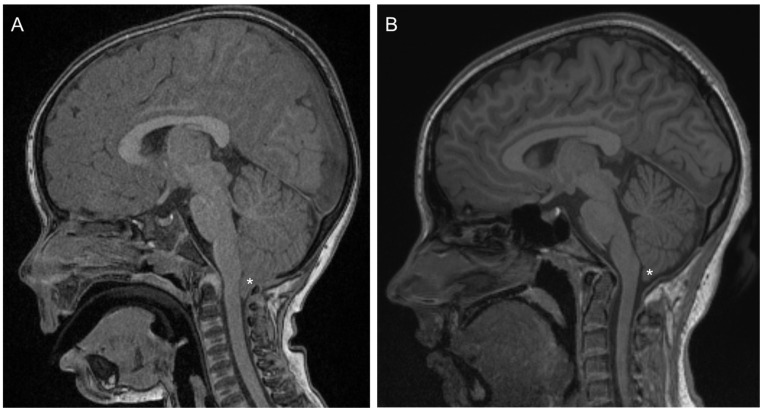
(A) Pre-operative T1-weighted sagittal MRI showing extent of herniation of the cerebellar tonsils (*) and associated compression of the cervico-medullary junction. (B) T1-weighted sagittal MRI collected 14 years after decompression surgery. The cerebellar tonsils (*) remain herniated by ~6.3 mm.
